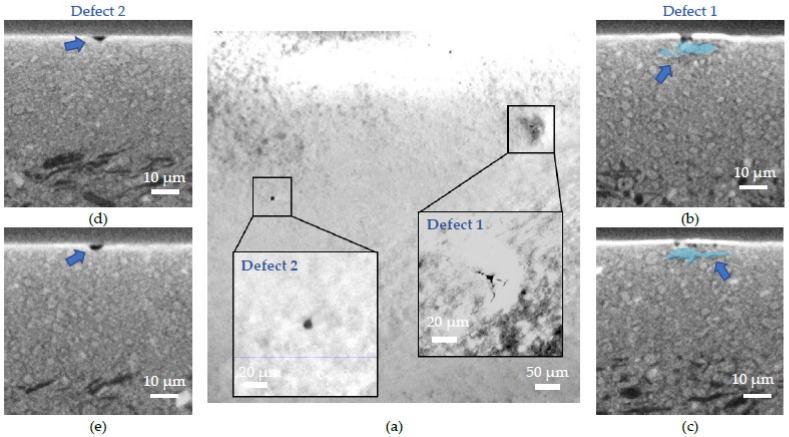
X-ray tomography images of defects in the annealed sample: (a) image along the *Y*-axis with focus on two defects, the inserts show higher magnification images of the defects; (b) cross-section of Defect 1 along the *X*-axis; (c) cross-section of Defect 1 along the *Z*-axis; (d) cross-section of Defect 2 along the *X*-axis; (e) cross-section of Defect 2 along *Z*-axis.
